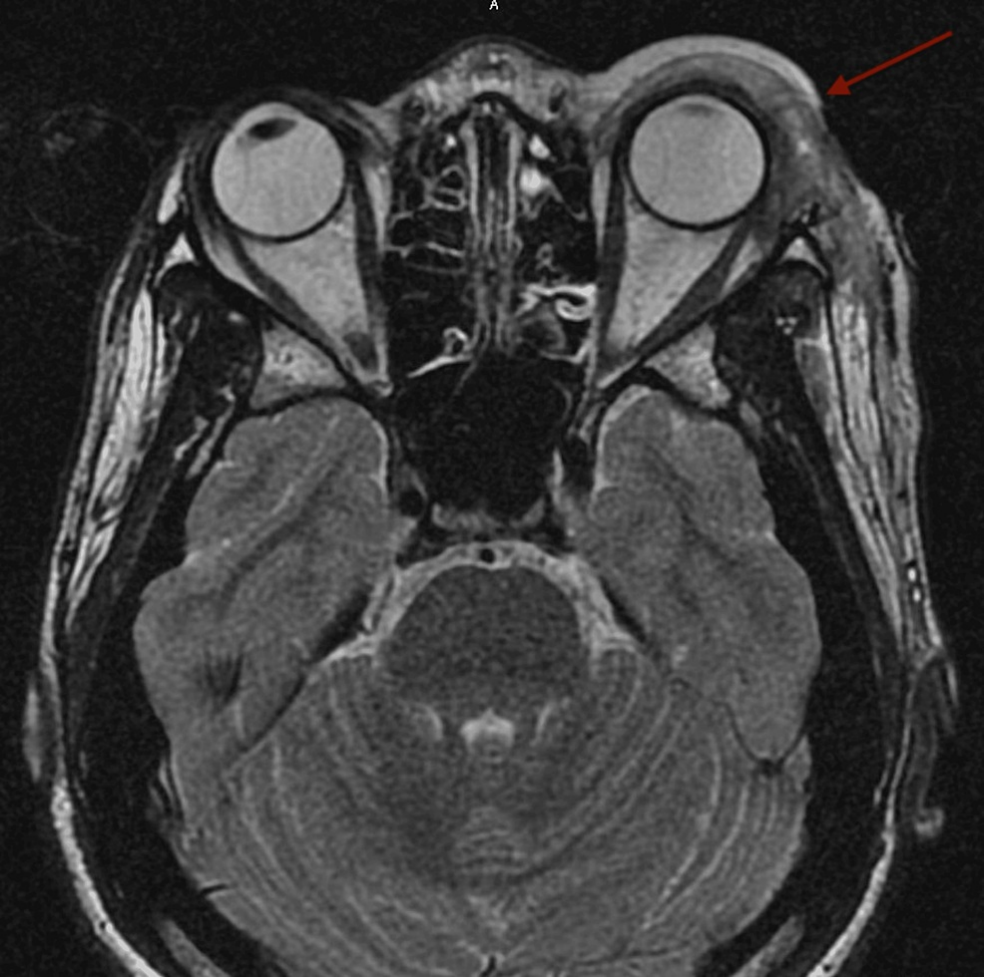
Axial T2 FLAIR magnetic resonance imaging of the orbits showing bony irregularity, edema, and erosions of the superolateral orbital rim consistent with osteomyelitis and post-septal inflammation of the left lateral and superior rectus muscles FLAIR: fluid-attenuated inversion recovery
